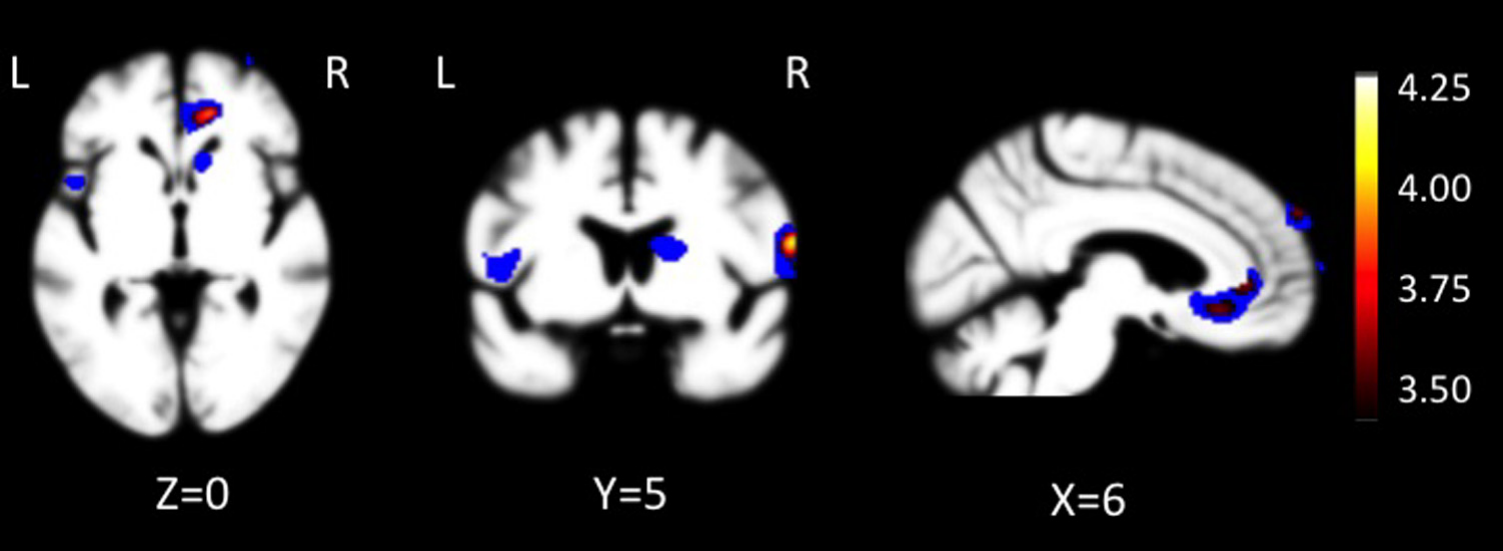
Neural substrates of BIS score. T-score maps of brain areas for which larger volume is associated with higher BIS score when controlling for age, education, gender, type of MRI scan, and total intracranial volume. Areas include right anterior cingulate, right precentral gyrus, right middle frontal gyrus, left dorsal anterior insula/operculum, and right superior frontal gyrus (pFWE < 0.05). Color bar represents T-scores (hot, *T* = 3.72, pFWE < 0.05 according to study-specific permutation analysis; blue, *P *<* *0.001, uncorrected; *T* > 3.18 and cluster size >150 mm^3^). Images were overlaid with MRIcron (http://www.mccauslandcenter.sc.edu/ CRNL/) on an average brain based on the healthy older control gray matter template used for DARTEL warping.
